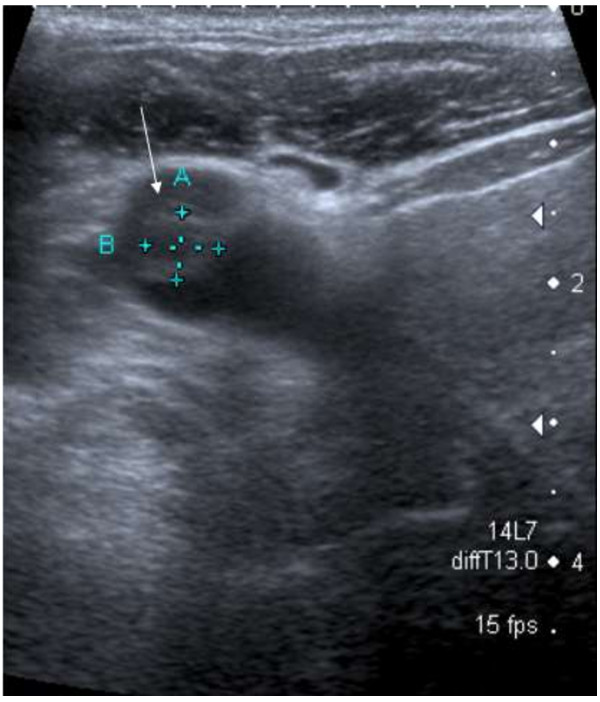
>50% occlusive right internal jugular vein thrombus on ultrasonography.
